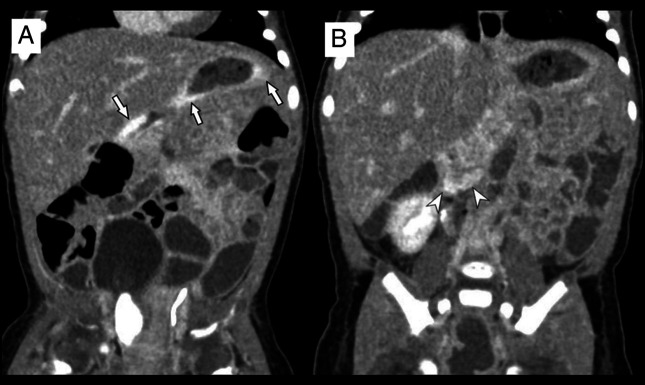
Abdominal computed tomography images in coronal plane showed multiple enhancing lesions in the gastric mucosa (arrows, A) and contrast extravasation in the gastric antrum (B).
